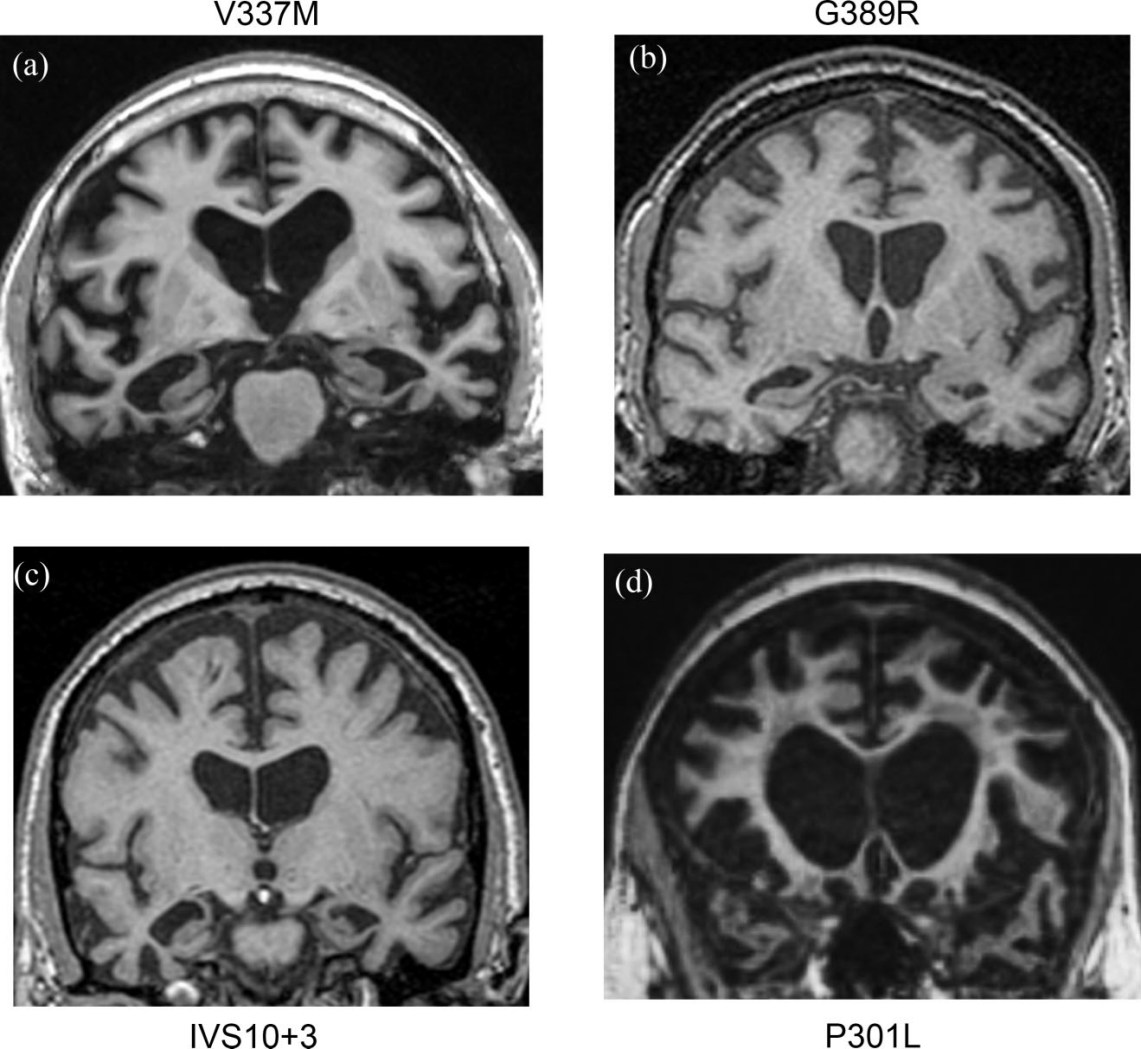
Coronal T1-weighted magnetic resonance imaging (MRI). Panel a is from a 65-year-old male with behavioural-variant frontotemporal dementia associated with the V337M *MAPT* mutation. Symptoms evolved over 20 years. Note the moderate to marked bilateral frontal and temporal cortical atrophy, with a severe anterior temporal lobe atrophy. Panel b is from a 25-year-old male with frontotemporal dementia and primary progressive aphasia associated with the G389R *MAPT* mutation. Symptoms rapidly developed over 1 year. Note the mild bilateral frontal and temporal cortical atrophy, with more pronounced medial and inferolateral anterior temporal atrophy. Panel c is from a 51-year-old male with behavioural-variant frontotemporal dementia associated with the IVS10+3 *MAPT* mutation. Symptoms evolved over 3 years. Note the mild bilateral frontal and temporal cortical atrophy with more pronounced mesial temporal atrophy. Panel d is from a 62-year-old female with severe behavioural-variant frontotemporal dementia associated with the P301L *MAPT* mutation. Symptoms evolved over 6 years. Note the striking bilateral prefrontal and anterior temporal atrophy with white matter changes.
